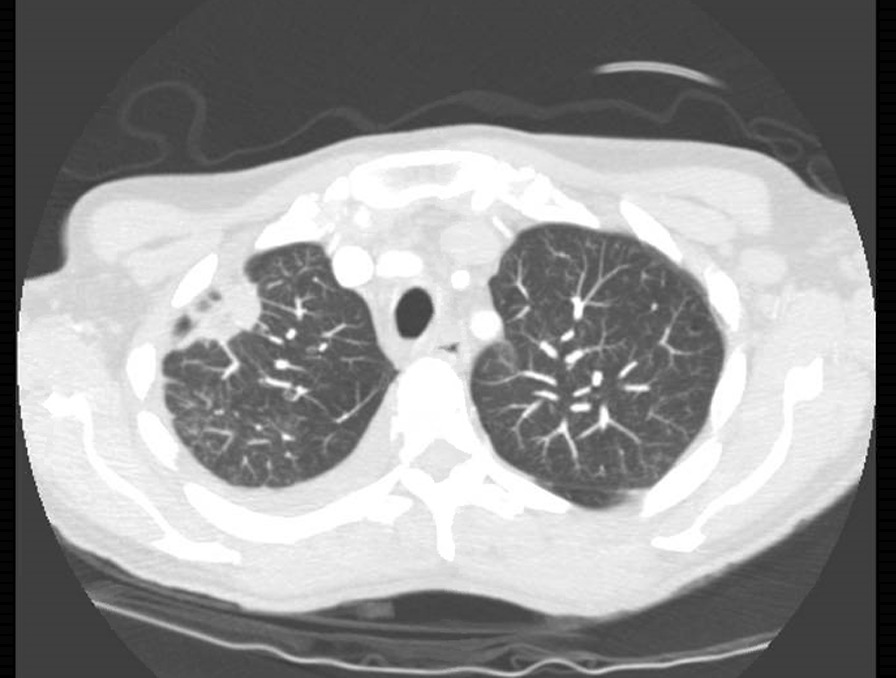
Computed tomography scan showing right upper lobe speculated anterior subpleural lesion extending on the anterolateral pleural surface, suggesting primary bronchial cancer
